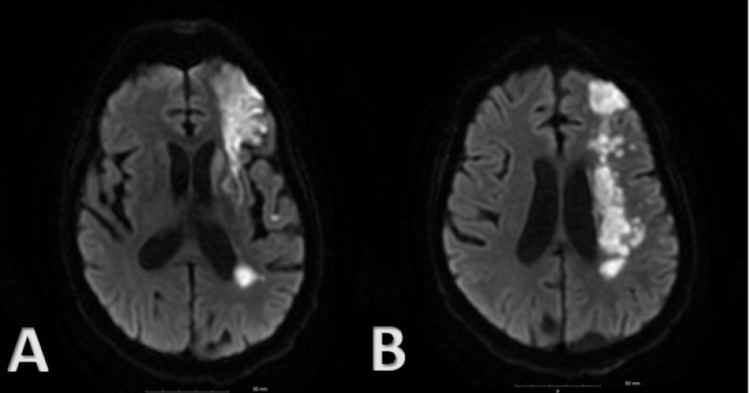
(A) MRI brain: DWI at the level of the basal ganglia. (B) MRI brain: DWI at the level higher than the basal ganglia demonstrating increased DWI signal in the left frontoparietal region consistent with acute watershed infarct. MRI: magnetic resonance imaging; DWI: diffusion-weighted imaging
